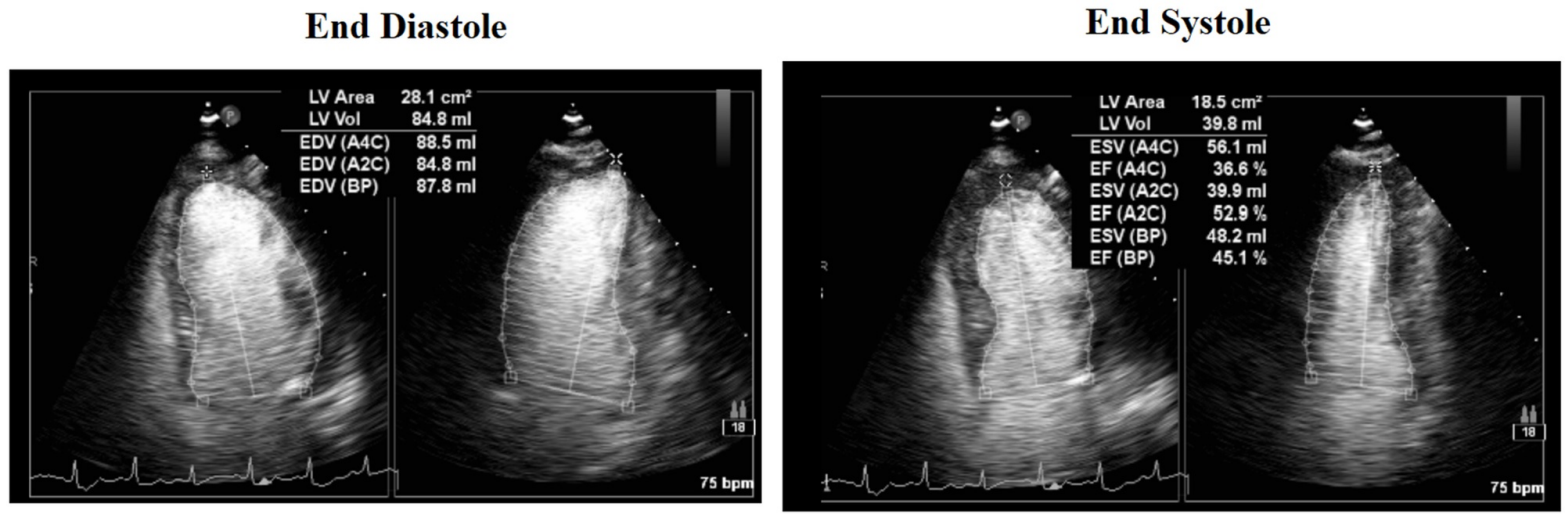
Cardiac echocardiogram End diastole versus end systole views: ejection fraction of 37%, moderate global hypokinesis.
